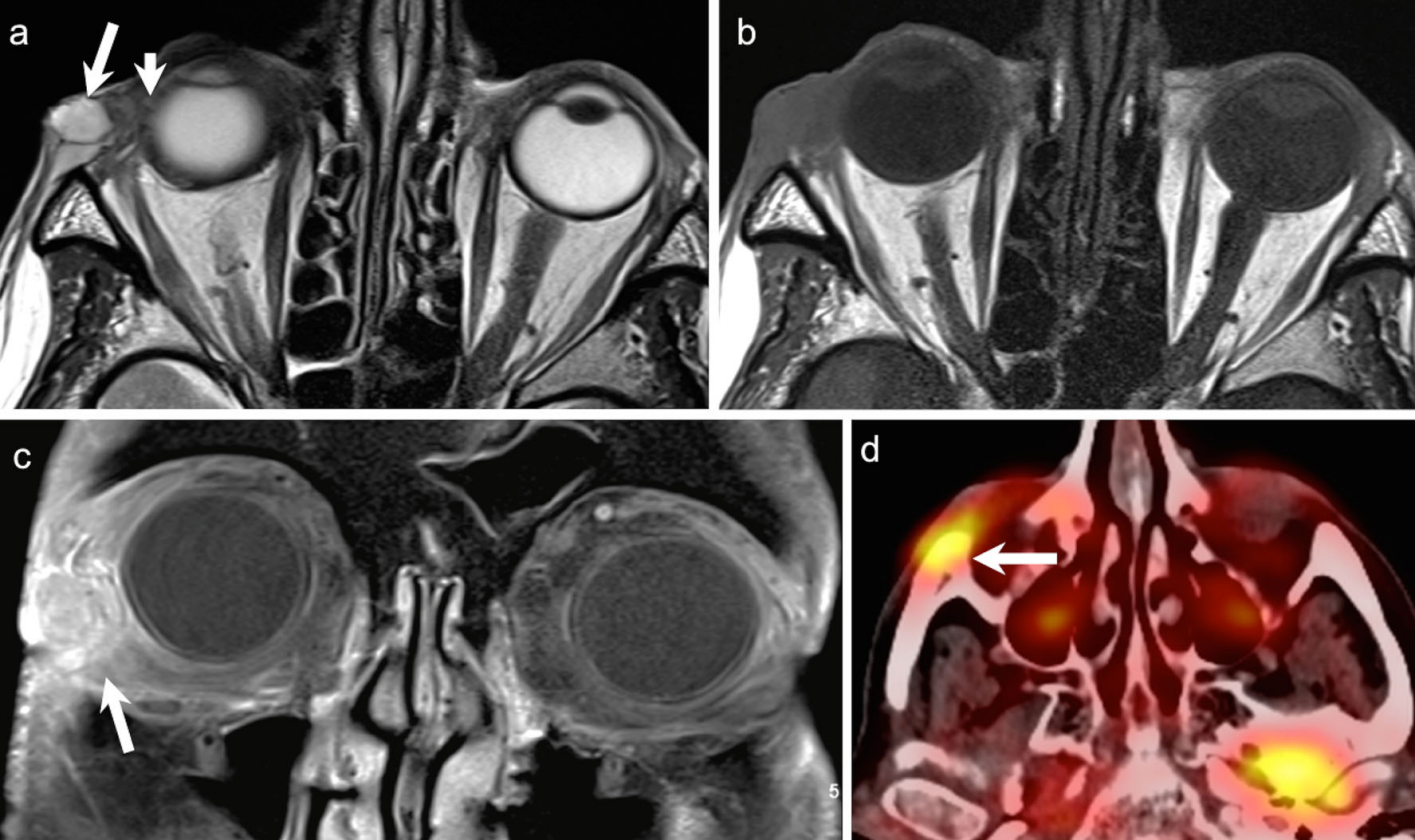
60-year-old male patient with desmoplastic melanoma of the right eyelid. a. Axial T2W image shows a heterogeneous signal-intensity lesion involving the subcutaneous tissue of the right lateral canthus (big arrow) with an infiltrating component extending into the extraconal compartment of the right orbit. There is suspicious invasion of the muscle cone and the globe (short arrow). b: Axial T1W image of the same patient does not show hyperintense signal within the lesion in keeping with low melanin content. c. Coronal contrast-enhanced FS TIW image reveals avid enhancement within the lesion. d. Axial PET/CT image of the same patient shows high FDG uptake within the lesion due to high glucose metabolism. No other lesions were detected in the rest of the body
